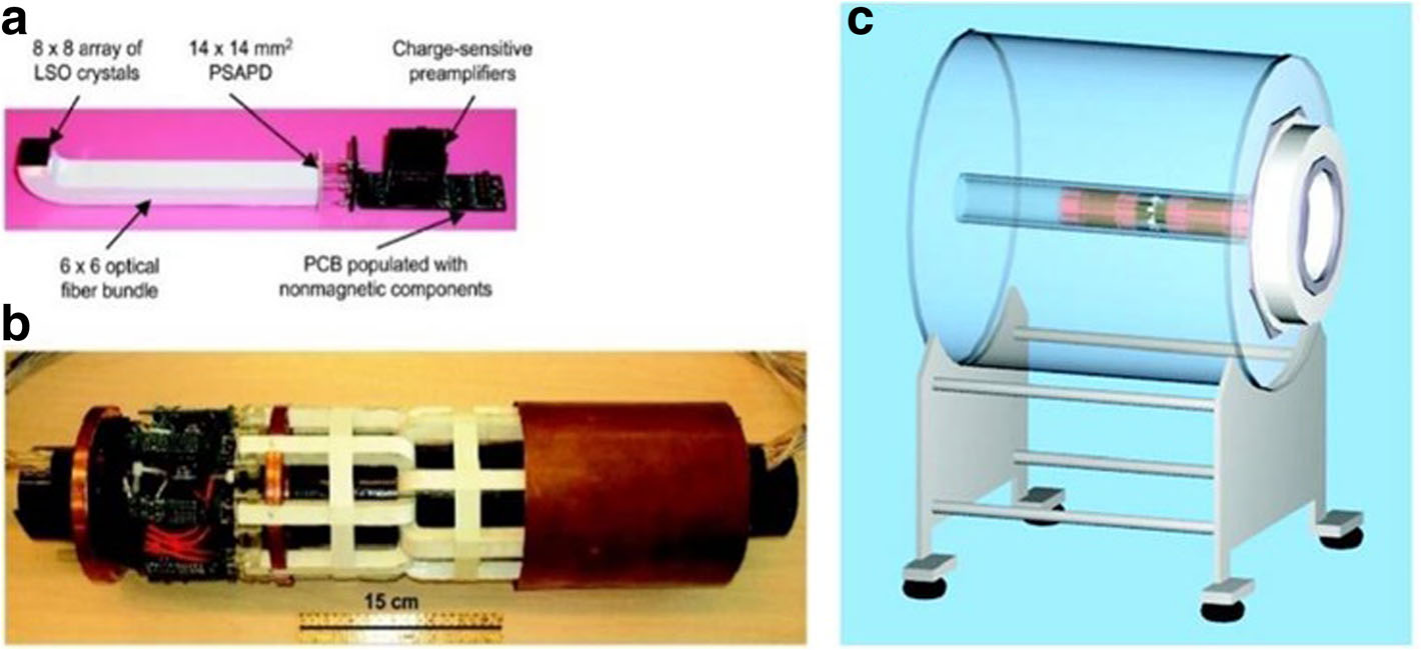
Shows (a) MR-compatible PET detector module, (b) complete MR-compatible PET scanner with 16 detector modules, shielding material (removed on left side), and carbon fibre tube for support and (c) Axial placement of PET insert inside 7 T MRI scanner (This research was originally published in *C. Catana, Y. Wu, M. S. Judenhofer, J. Qi, B. J. Pichler, and S. R. Cherry*, “Simultaneous Acquisition of Multislice PET and MR Images: Initial Results with a MR-Compatible PET Scanner, (Catana et al. 2006)” *J. Nucl. Med.*, vol. 47, no. 12, pp. 1968–1976, 2006.© by the Society of Nuclear Medicine and Molecular Imaging, Inc.)
